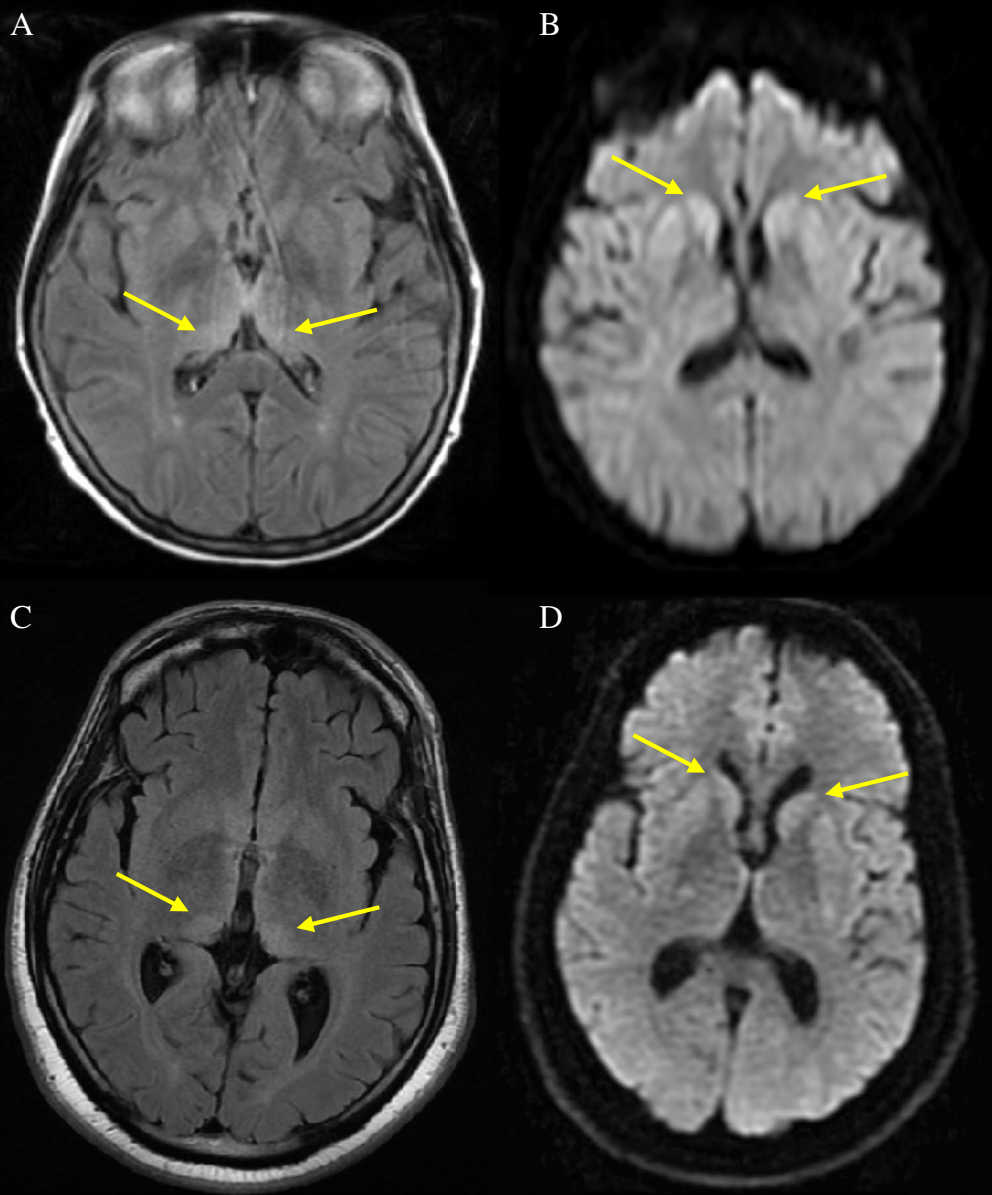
MRI findings in two neuropathologically confirmed cases of vCJD(A+B) and (C+D) Axial FLAIR images in both cases (A and C) demonstrating positive pulvinar signs, and associated dorsomedial thalamic high signal, with the corresponding DWI (B and D respectively) showing more conspicuous high signal in the basal ganglia in the absence of clear pulvinar signal abnormality
